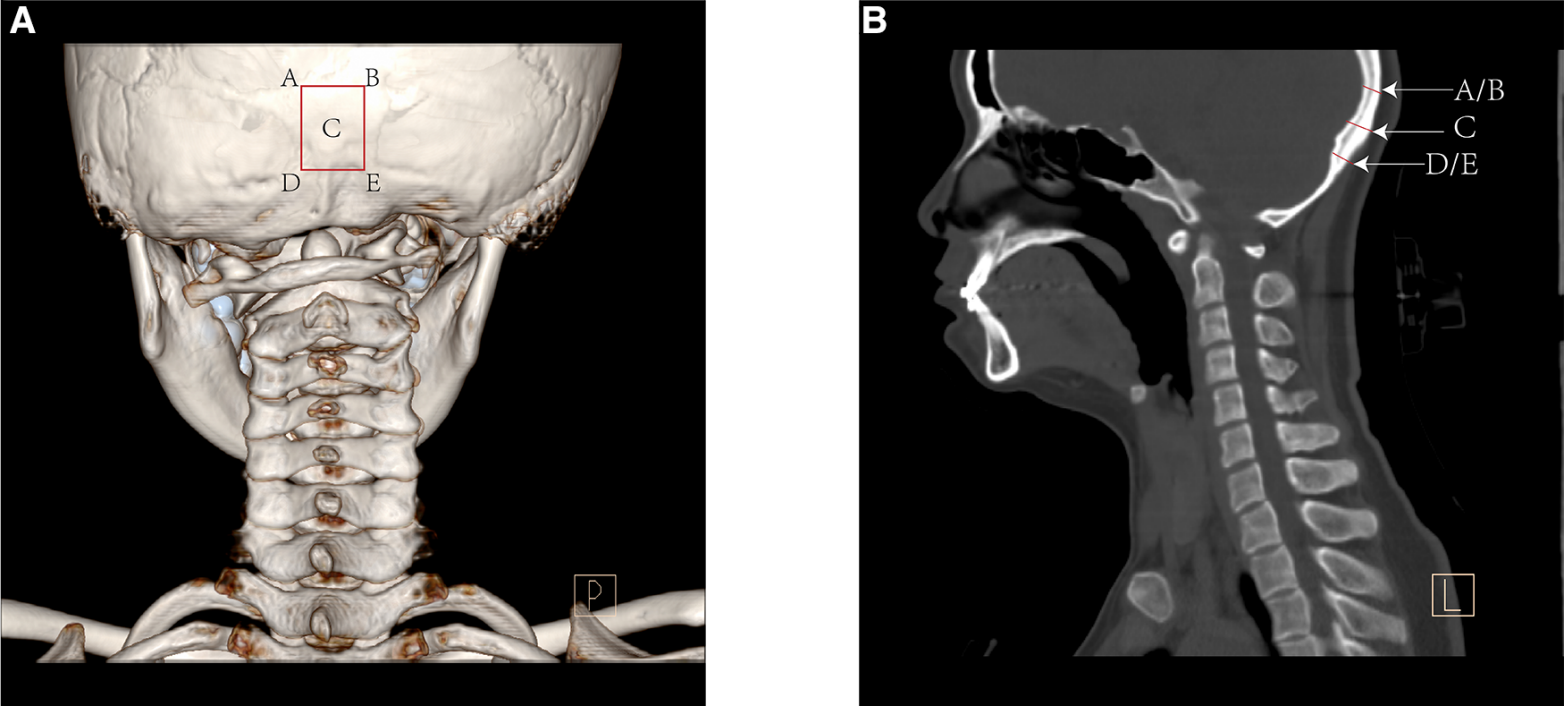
The posterior view of computed tomography (CT) 3D reconstruction (A) and the sagittal reconstruction (B) showed the method to measure the bone thickness of the occipital donor-site.
